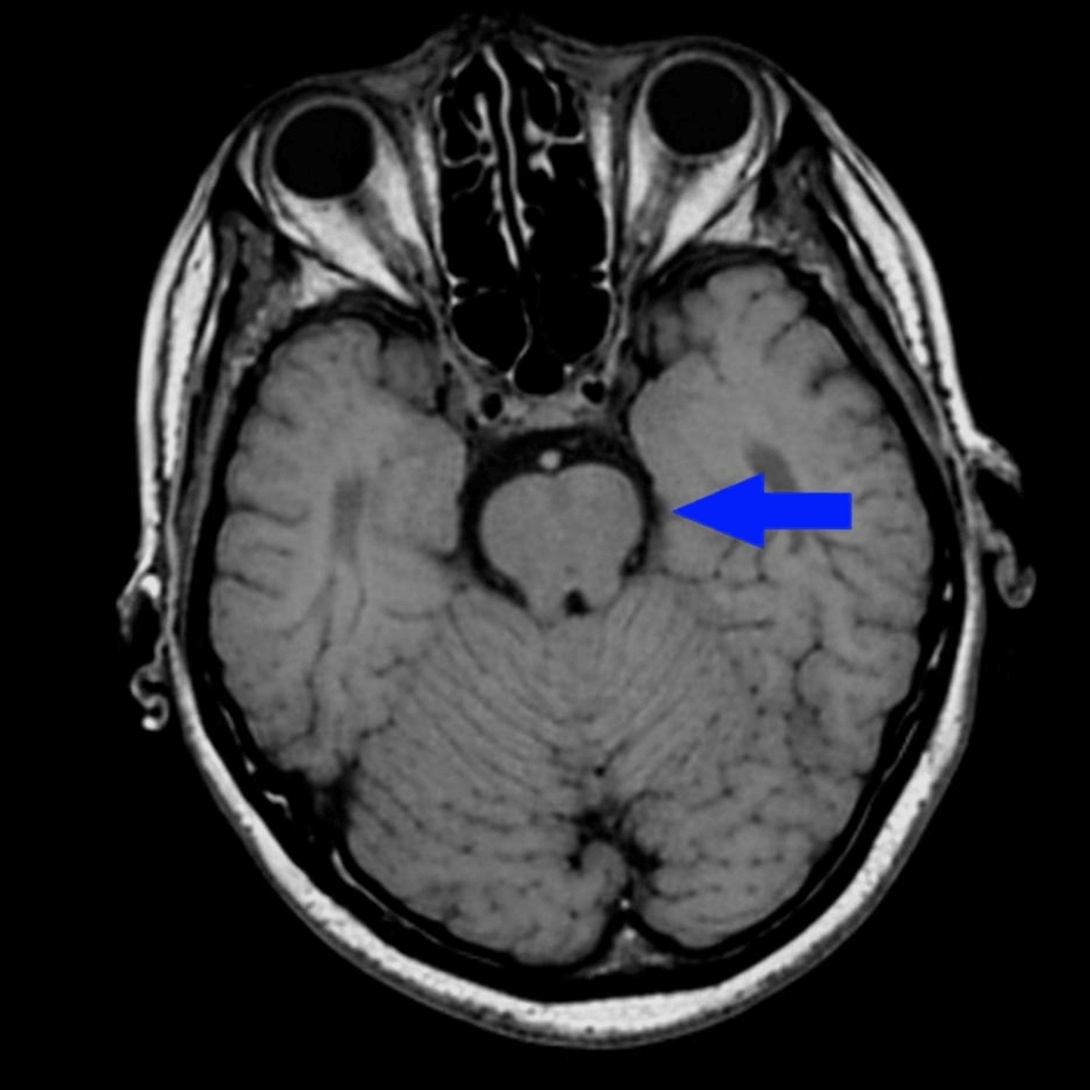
Brain imaging performed approximately four days after return of spontaneous circulation, after hemodynamic stability permitted transport, demonstrates no acute diffusion restriction. The blue arrow corresponds to the same left cerebellar region previously identified on CT (Figure 1), where no corresponding lesion is visualized on follow-up imaging.
